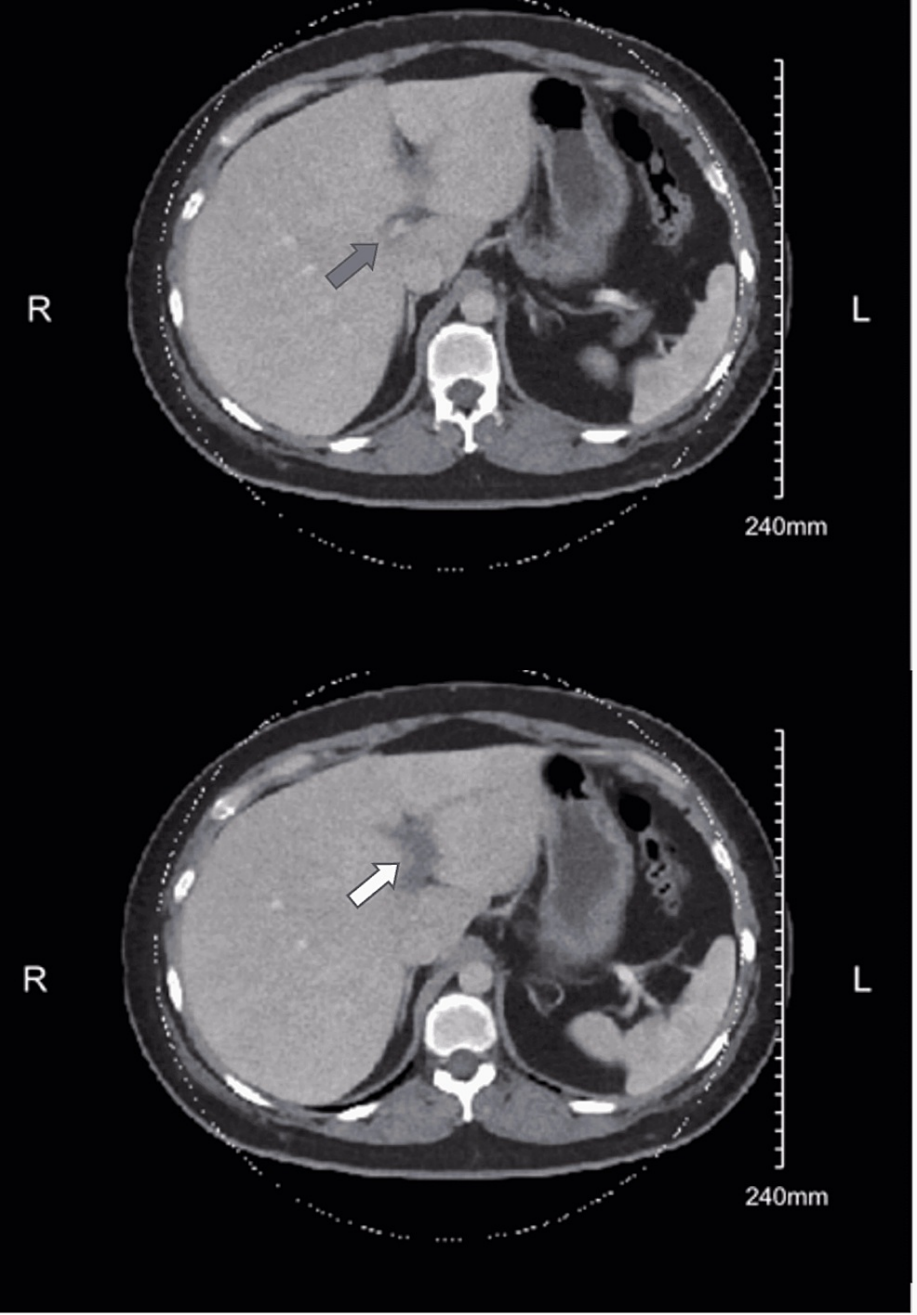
CT abdomen (axial view) Axial view of proximal (grey arrow) and distal portal vein thrombosis (white arrow)
